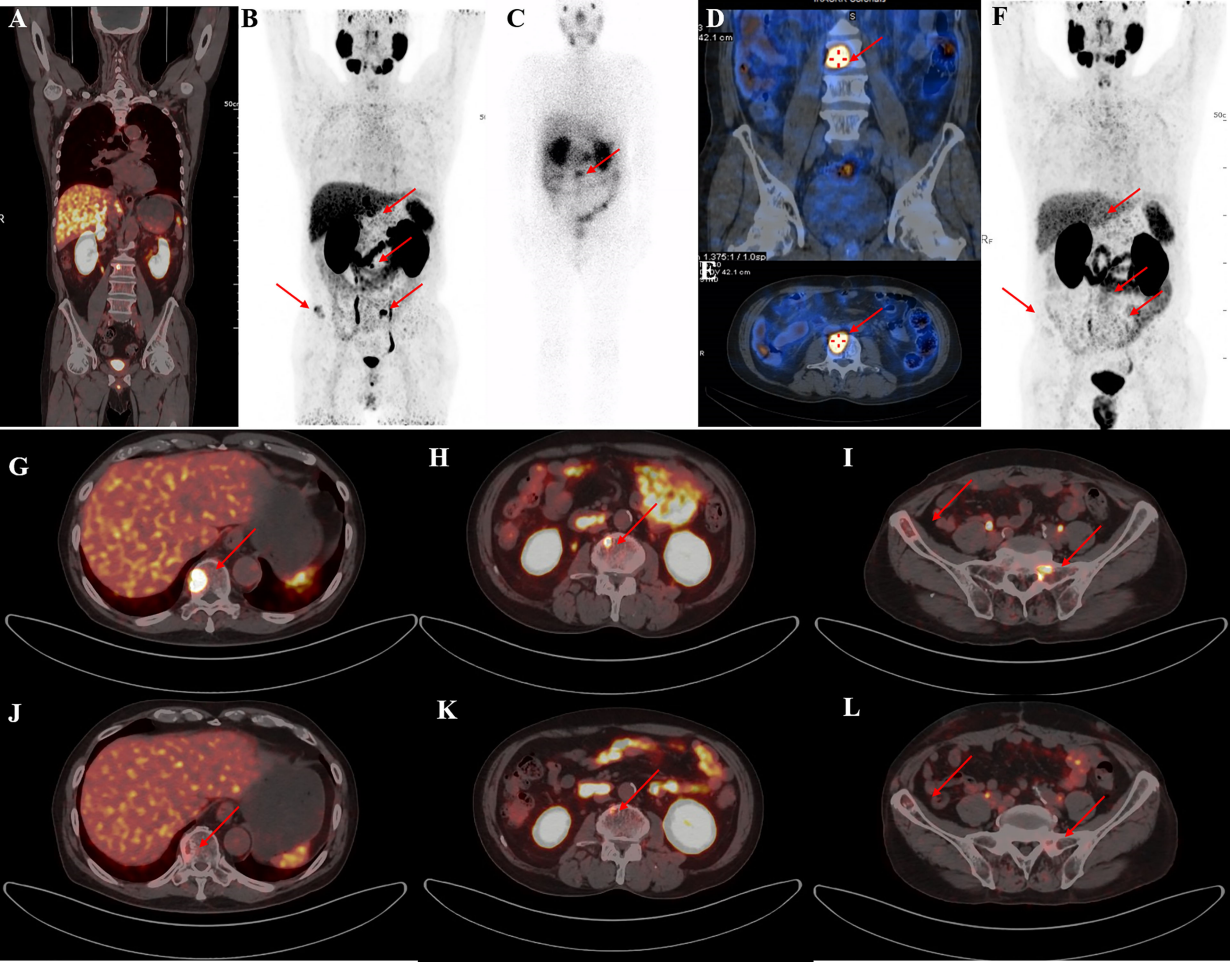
A representative 59-year-old patient with PSA decline ≥99% before and after Lu-PSMA-I&T therapy. (A, B) Baseline image and (F) image 3 months post-therapy. ^177^Lu-PSMA SPECT whole-body scan (C) showing higher contrast images 24 h post-injection with significant accumulation in the tenth thoracic vertebra sclerotic lesion. (D, E): ^177^Lu SPECT image at 48 h showing destroyed and uneven density of L3 vertebrae with PSMA-avid lesion. Fusion image (G-L) showing reduced metastases in T10, L3, the right iliac, and sacrum compared with before therapy.
